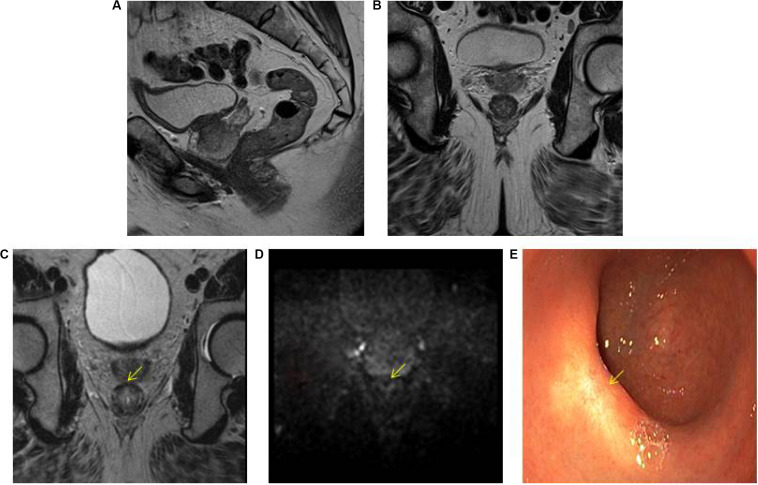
Three-modality approach with combination of T2W-MRI, DWI, and endoscopy of the selection of a patient with a clinical complete response. A low rectal tumor is seen on MRI (A, B). On restaging MRI 8 weeks after completion of chemoradiotherapy (C) only minimal fibrosis (yellow arrow) is seen anteriorly in the rectal wall. On restaging DWI (D) there is absence of high diffusion signal (yellow arrow). Clinical assessment by endoscopy (E) reveals a white scar with telangiectasia (yellow arrow).
